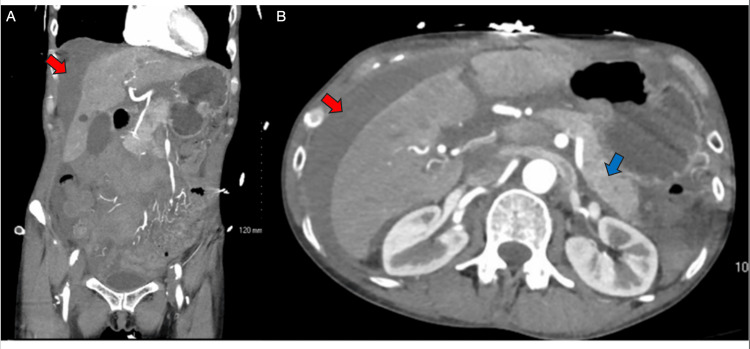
Day 2 repeat CTA of the abdomen and pelvis (multiplanar views). Repeat CTA on day 2 shows stable mesenteric hematoma with faint hyperattenuation in the right upper abdomen, likely due to hyperemia or resolving extravasation. No new active bleeding is identified. Duodenal and jejunal wall thickening is noted, suggestive of enteritis (A) Coronal view: stable mesenteric hematoma with faint hyperattenuation in RUQ (red arrow); no new extravasation. (B) Axial view: stable hematoma (red arrow) with duodenal and jejunal wall thickening (blue arrow), suggestive of enteritis. CTA: computed tomography angiogram; RUQ: right upper quadrant
